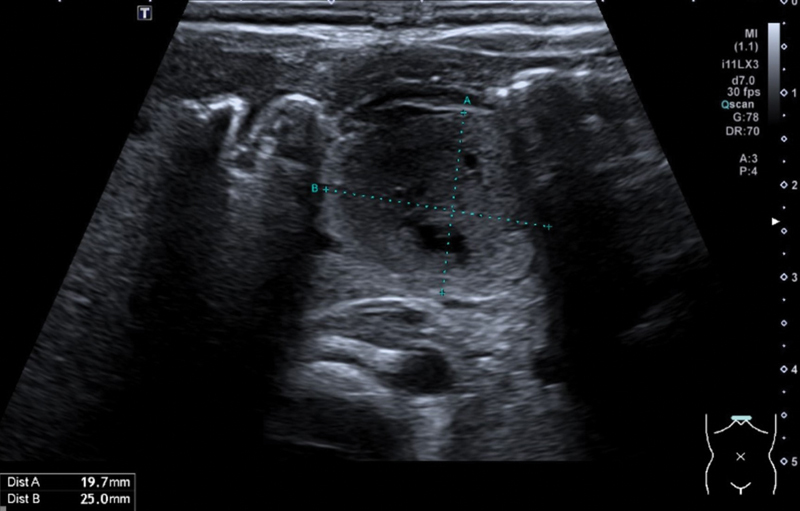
Preoperative ultrasound showing the mass close to the stomach, pancreas, and duodenum.
